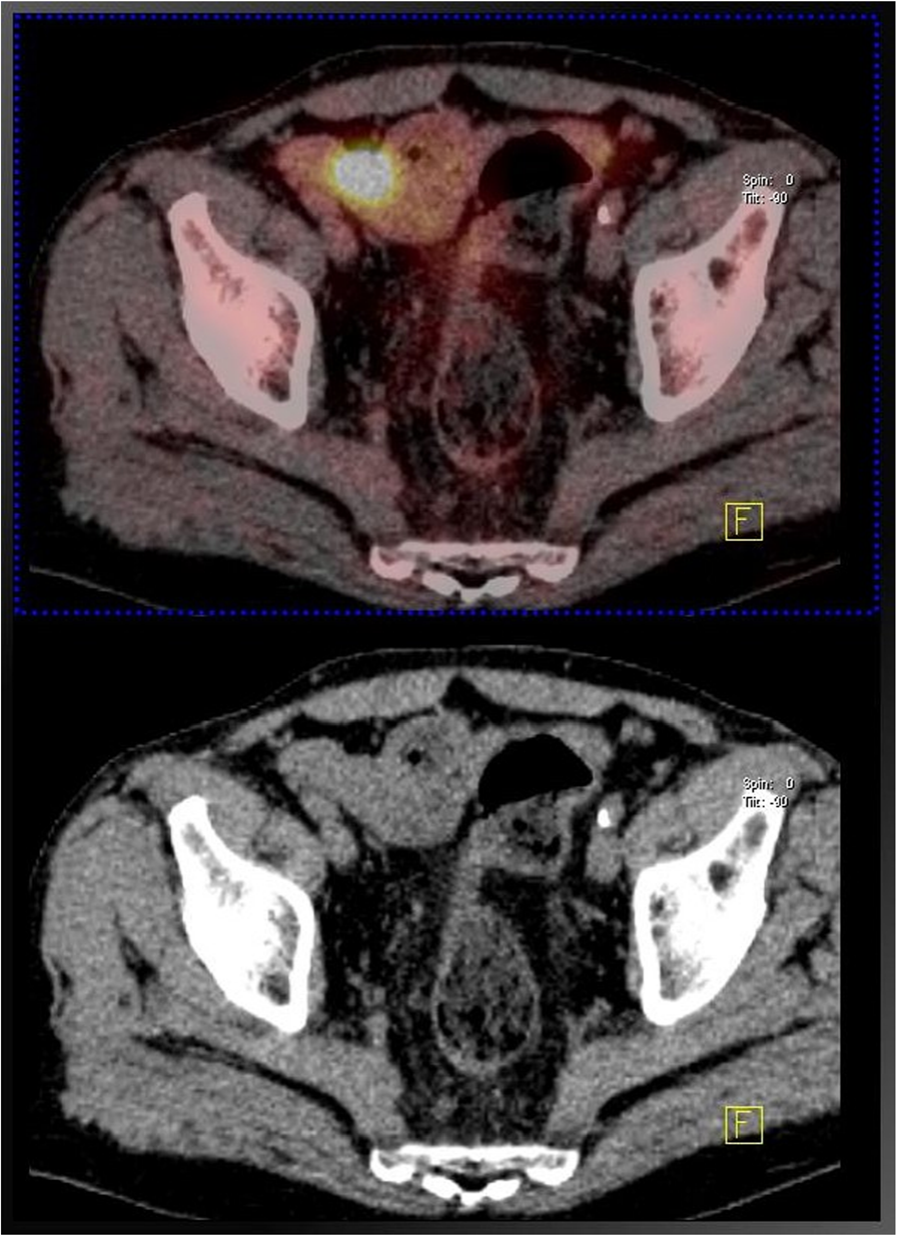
PET/CT incidental detection of cancer of the sigmoid colon 39 months after pancreatico-duodenectomy for pancreatic cancer
